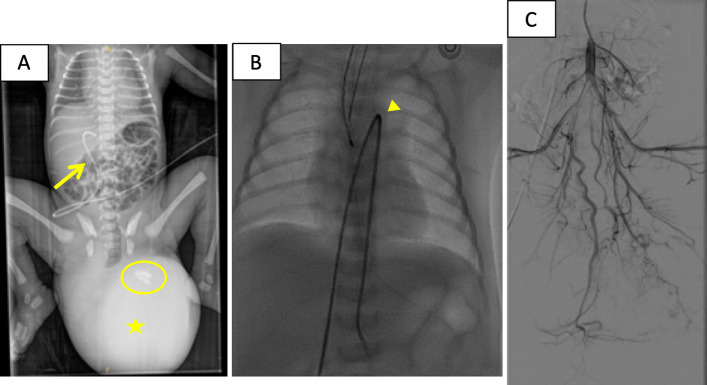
Abdominal radiograph on the first day of life (A) highlights a soft tissue mass caudal to the sacrum (star), with coarse calcification within it (circle), corresponding to the SCT. The patient has an umbilical vein already cannulated (arrow) by the pediatricians. Fluoroscopy image (B), of the catheter crossing through the ductus arteriosus (arrowhead) to the descendent aorta. Arteriography (C) from the aortoiliac bifurcation, identifying profuse vascularization of the SCT dependent on a hypertrophic middle sacral artery and branches of both internal iliac arteries
